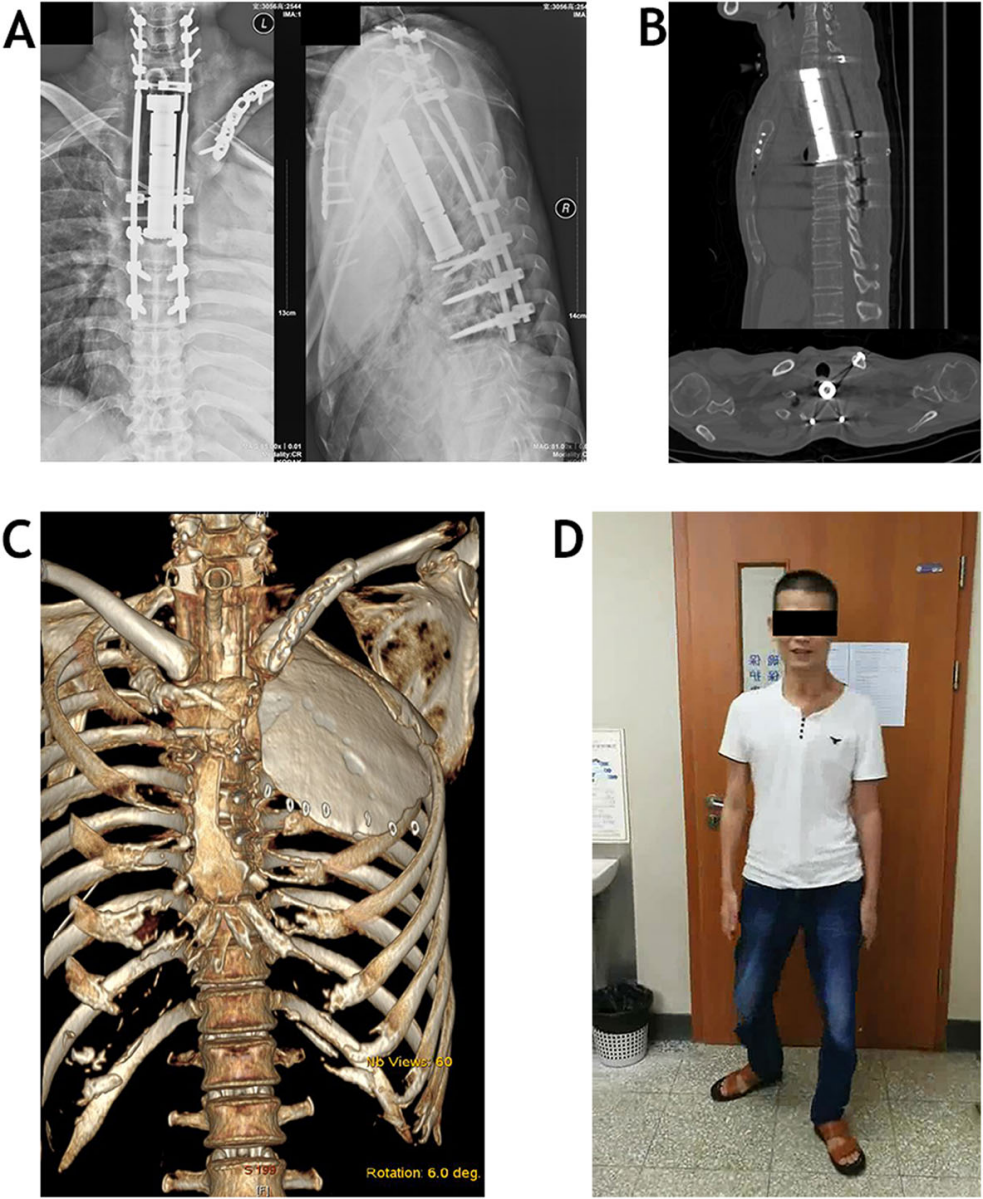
Postoperative plain radiograph (a), sagittal and axial view of computed tomography (b) show spinal reconstruction and internal fixation of sternum and left clavicle. The sandwiched bone cement plate for chest wall repair displays on Three-dimensional computed tomography reconstructions (c). This patient who walked independently was followed up at clinic 6 months after operation (d)
